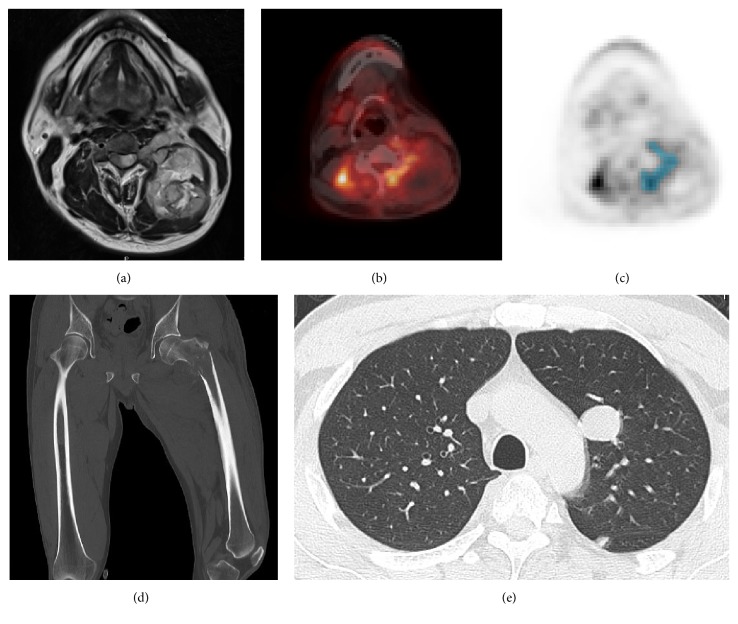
Representative case presentation of SUV*
